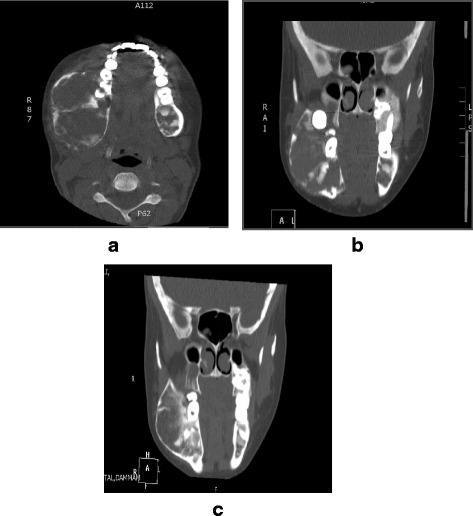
Maxillofacial computed tomographic scans with contrast enhancement showing multiple expansile lesions with ossification. The largest lesion can be seen within the body and ramus of the mandible. a Preoperative scan. b Scan obtained 5 months postoperatively demonstrating increased ossification of the lesion. c Scan obtained 1.5 years postoperatively showing reduction in the size of the lesion
